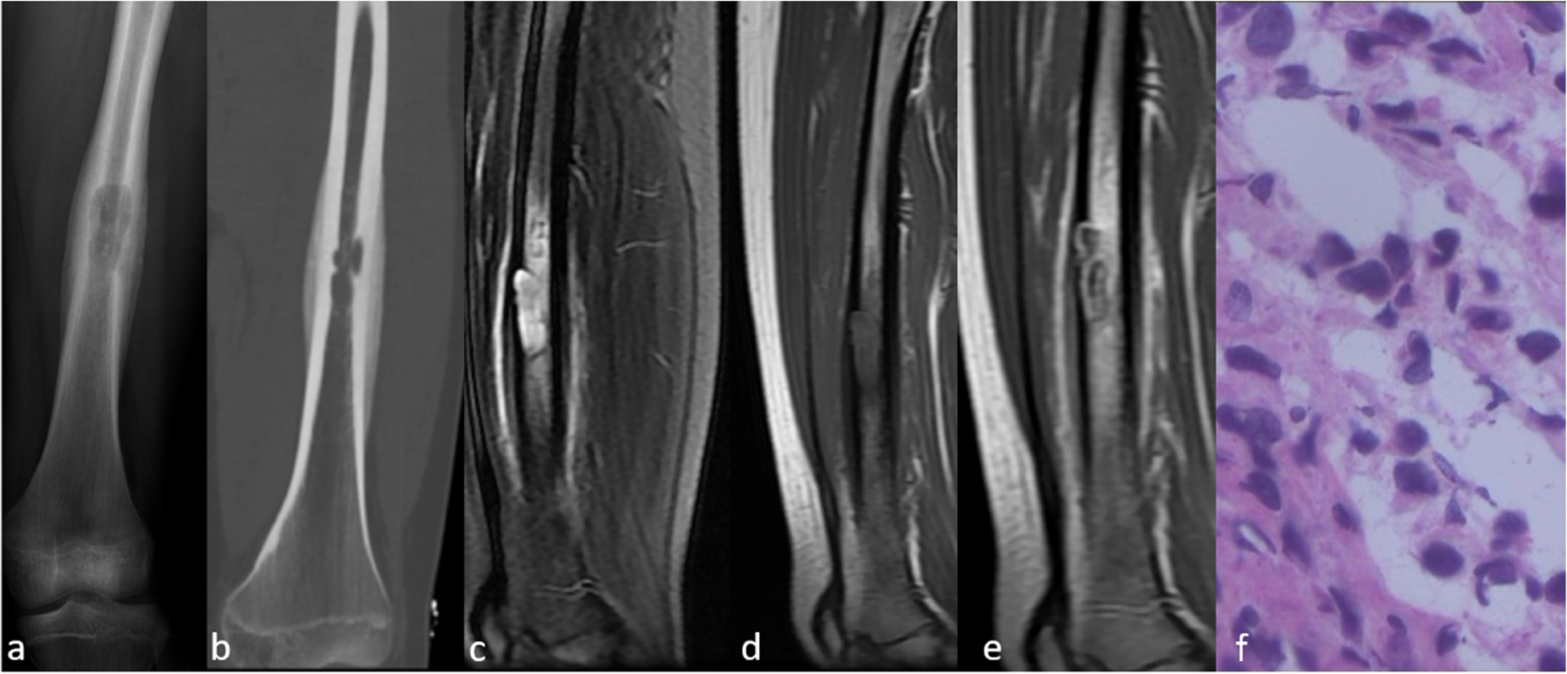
X-ray (a), CT (b), and MRI (c-e) of the left femur of a 12-year-old female patient. X-ray image (a) and CT (b) show an oval lesion in the middle left femoral diaphysis, associated with fusiform periosteal reaction. The lesion shows hyperintense signals and surrounding marrow and soft tissue oedema on FS-T2WI (c), hypointense signal on T1WI (d), and obvious enhancement after gadolinium administration. The diagnosis of LCH was confirmed by pathology after surgery (pathological slice HE × 400) (f)
